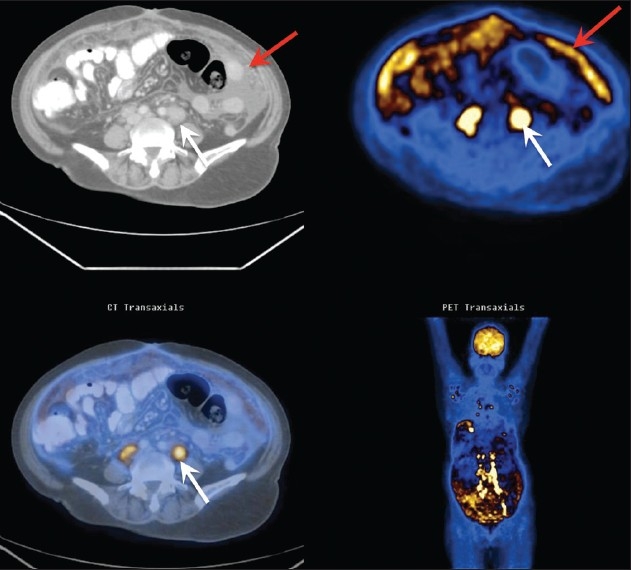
FDG-PET/CT demonstrates metabolically active retroperitoneal adenopathy (white arrows), ascites, metabolically active peritoneal deposits and extensive metabolically active omental thickening (red arrows)
